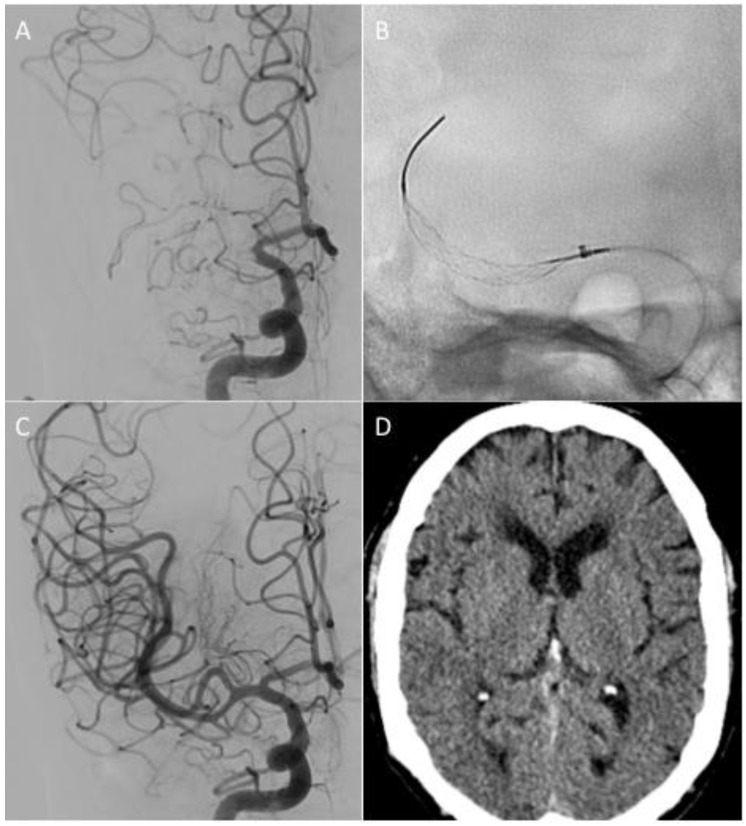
Angiography confirmed the terminal right ICA and M1 occlusion (A). The thrombus was crossed using a Headway 21 microcatheter, and a Stream device was expanded for 5 min (B). Withdrawal into the aspiration catheter was performed, during which the first-pass effect was observed (eTICI 2c) (C). Non-contrast CT scan of the head performed at 24 h demonstrated no evidence of haemorrhage and an ASPECT score of 8 (D).
